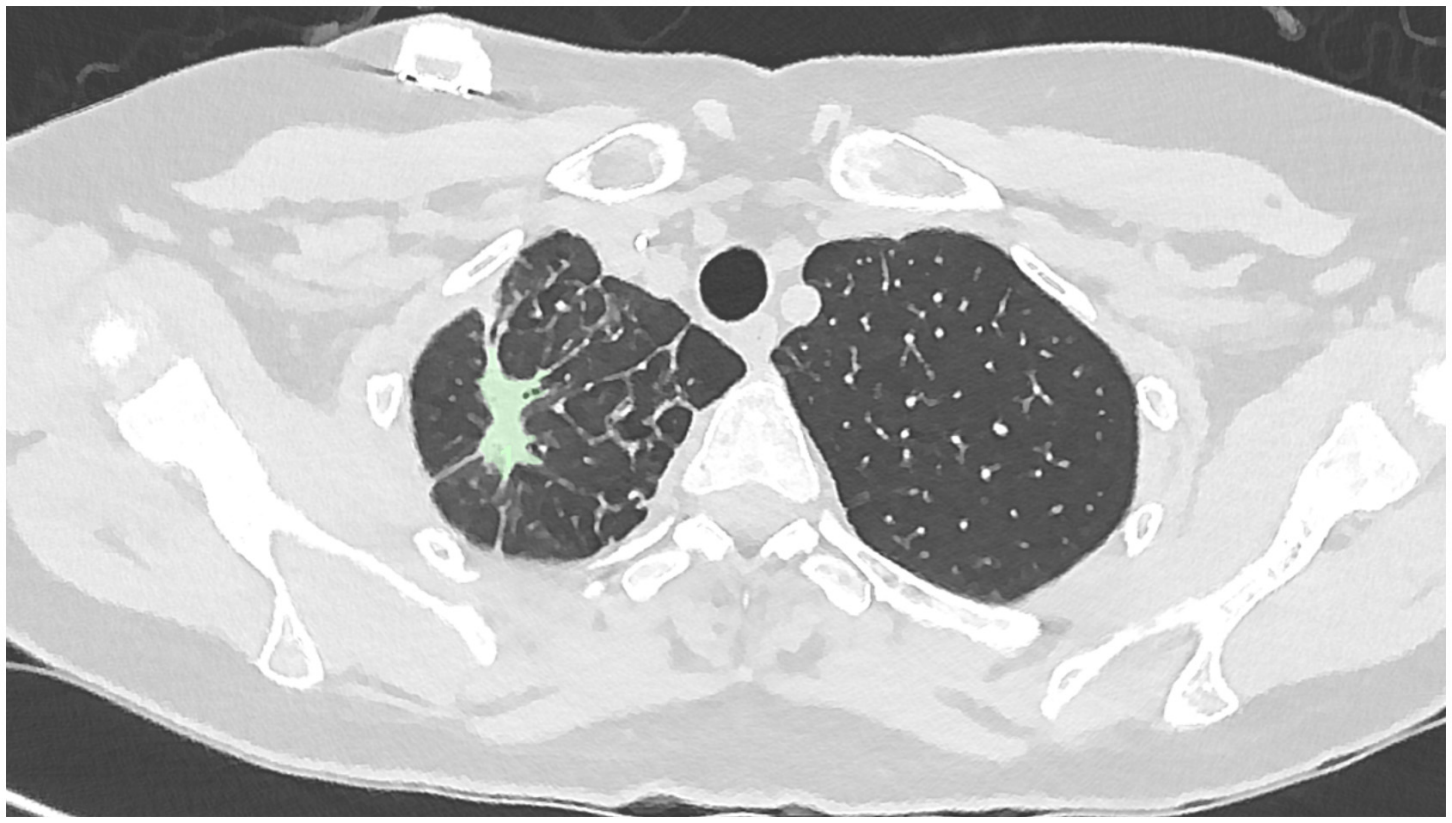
Low-dose CT chest at Day 64 post-treatment initiation demonstrating complete closure of the cavitary component with minimal residual parenchymal abnormality.
